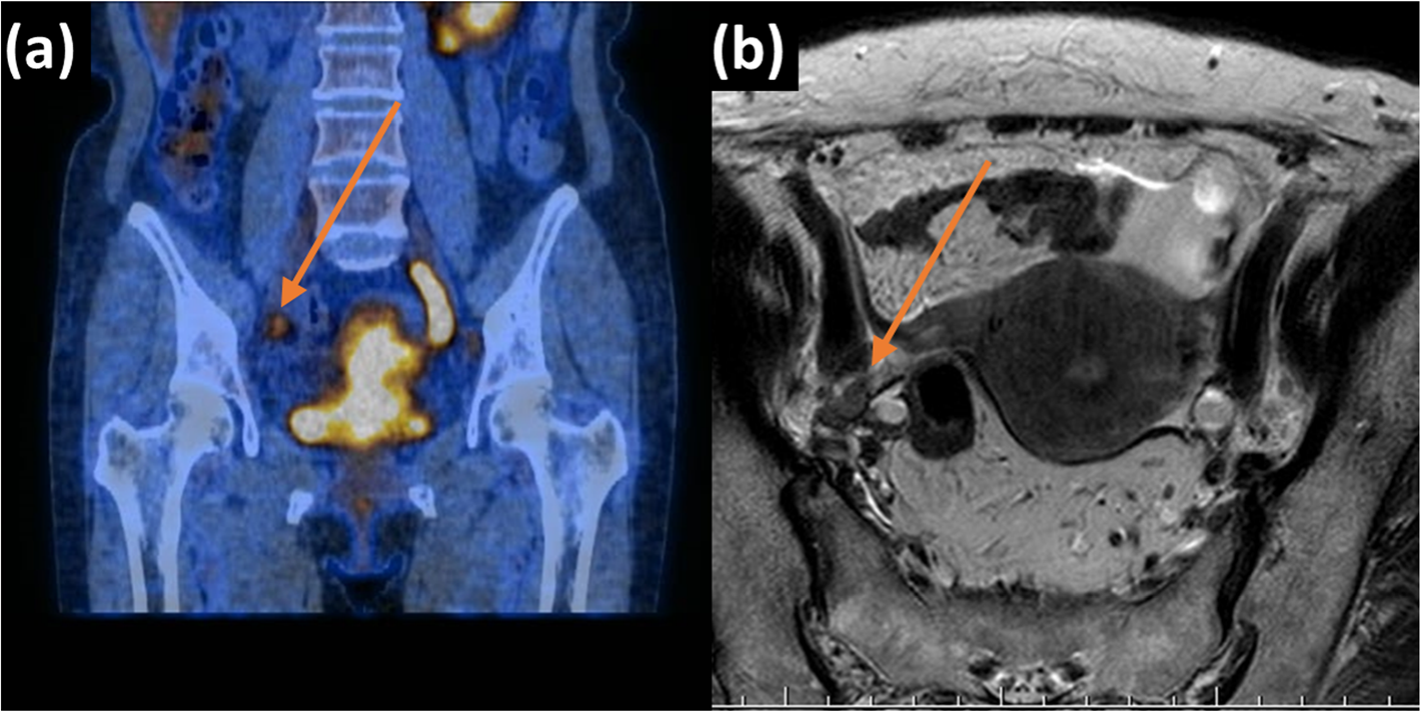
A case of a 67-year-old patient International Federation of Gynaecology and Obstetrics (FIGO) staged IIIB with a sub-centimetre pelvic lymph node on parametric maps of (a) coronal fused ^18^F-fluoro-deoxyglucose positron emission tomography and computed tomography (FDG-PET/CT) image and (b) axial T2-weighted image. This patient was classified as metastatic by the IVIM models and correctly staged by the subspecialist but not the non-subspecialist.
